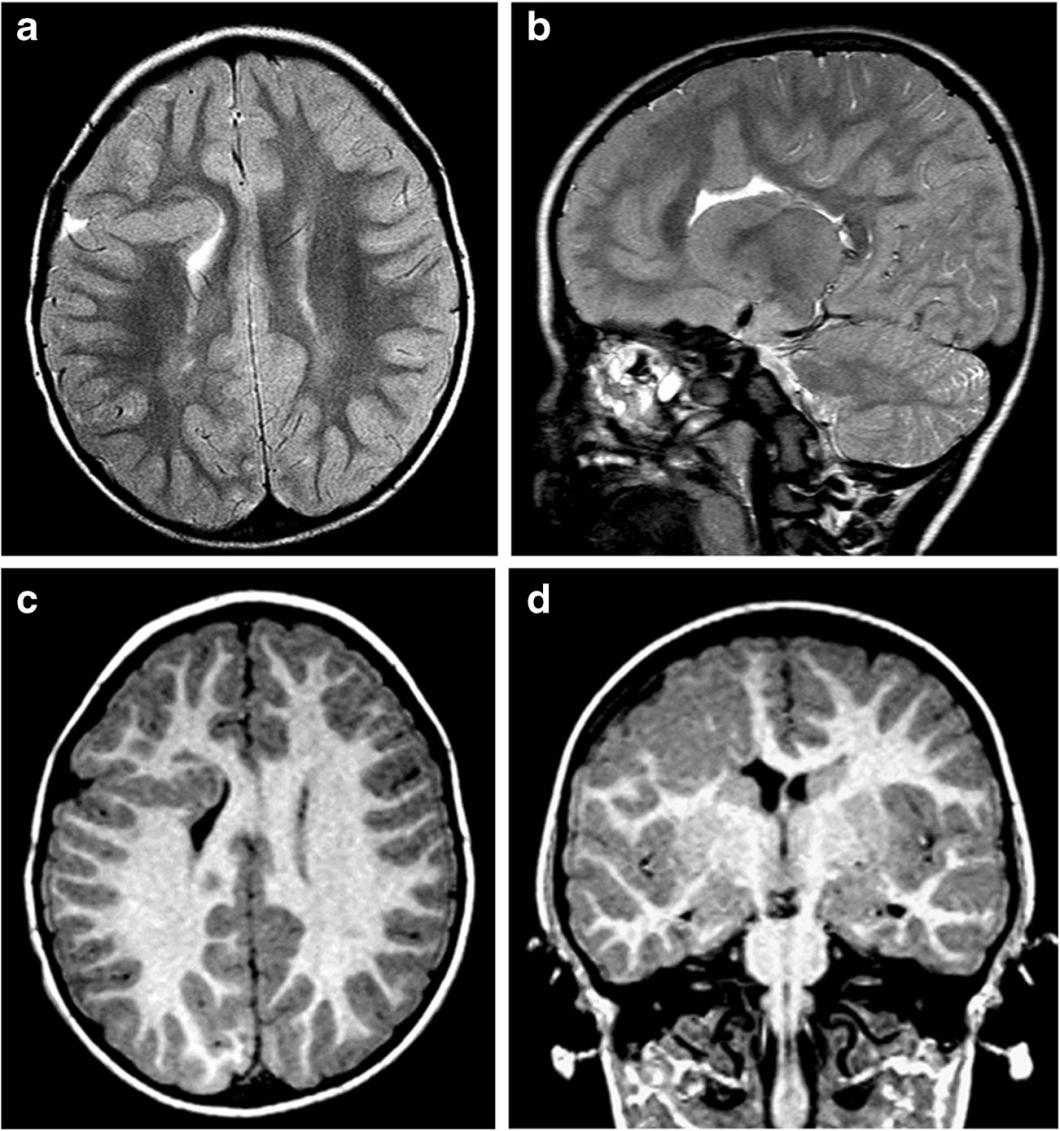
Unilateral schizencephaly (type 1). MR images of a 4-year-old child with focal epilepsy. Axial T2-weighted (a), right parasagittal T2-weighted (b), axial (c) and coronal reconstructions from T1 volume imaging show abnormal grey matter extending from ventricular to out surface of the brain, centred on the right middle frontal gyrus. No CSF cleft is visible, hence the classification as schizencephaly (type 1). The septum pellucidum is present and the course of the fornices is normal. No other brain abnormalities are present
